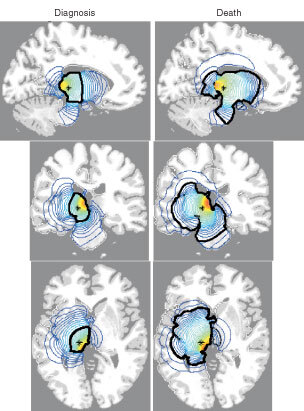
Sections of the virtual human brain in sagittal, coronal and horizontal planes that intersect at the site of the glioma originating in the thalamus denoted by an asterisk (*). The left column of brain sections corresponds to the tumour at diagnosis whereas the right column represents the same tumour at death. Red denotes a high density of tumour cells while blue denotes a low density. A thick black contour defines the edge of the tumour detectable by enhanced CT. Cell migration was allowed to occur in a truly three-dimensional solid representation of the brain. The elapsed time between diagnosis and death for this virtual gliomas is approximately 256 days.
